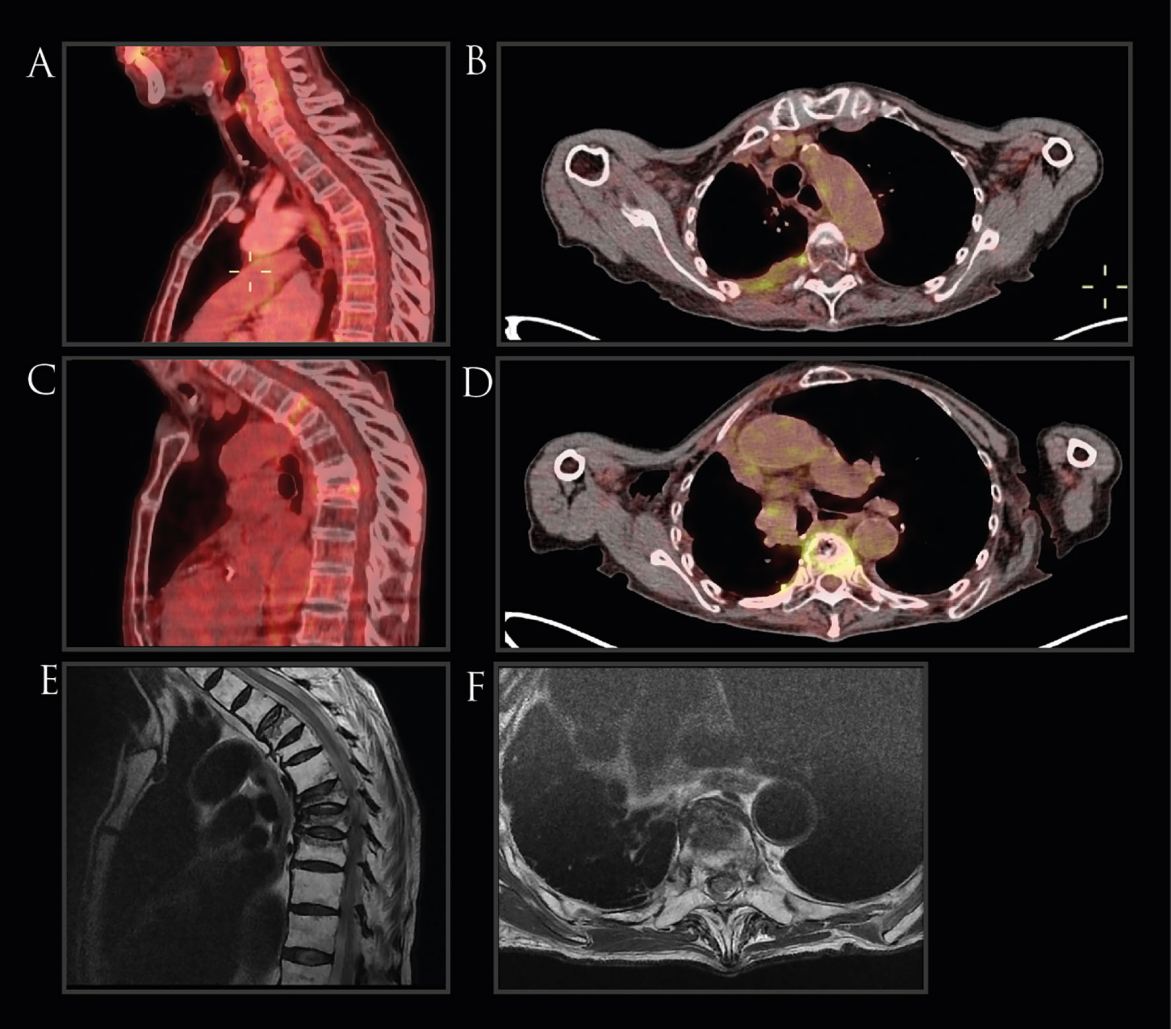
Imaging of Case 1. (A, B) PET-CT scans conducted prior to radiotherapy revealed no evidence of vertebral fractures. (C, D) Follow-up PET-CT scans post-radiotherapy, depicting compression fractures in the T4, T7, and T8 vertebrae, accompanied by diffuse metabolic activity, absent in prior imaging. (E, F) MRI showing the presence of vertebral fractures.
